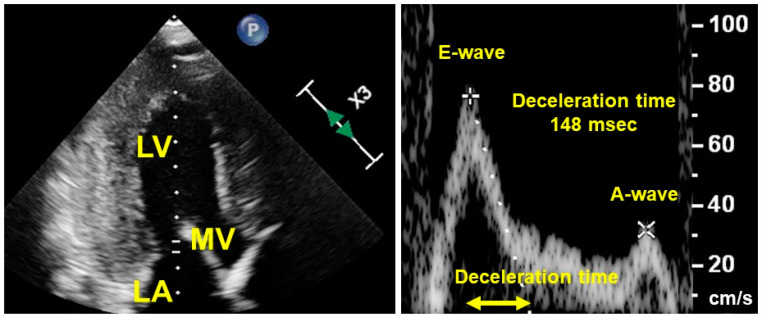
A representation of trans-mitral Doppler echocardiography, presenting a restrictive pattern consisting of a high and narrow E-wave and a small A-wave. LV, left ventricle; MV, mitral valve; LA, left atrium. The patient had heart failure with preserved ejection fraction due to cardiac amyloidosis. The deceleration time of this patient was short. A relatively higher heart rate may be required for both waves to stand adjacent and maximize cardiac output.
